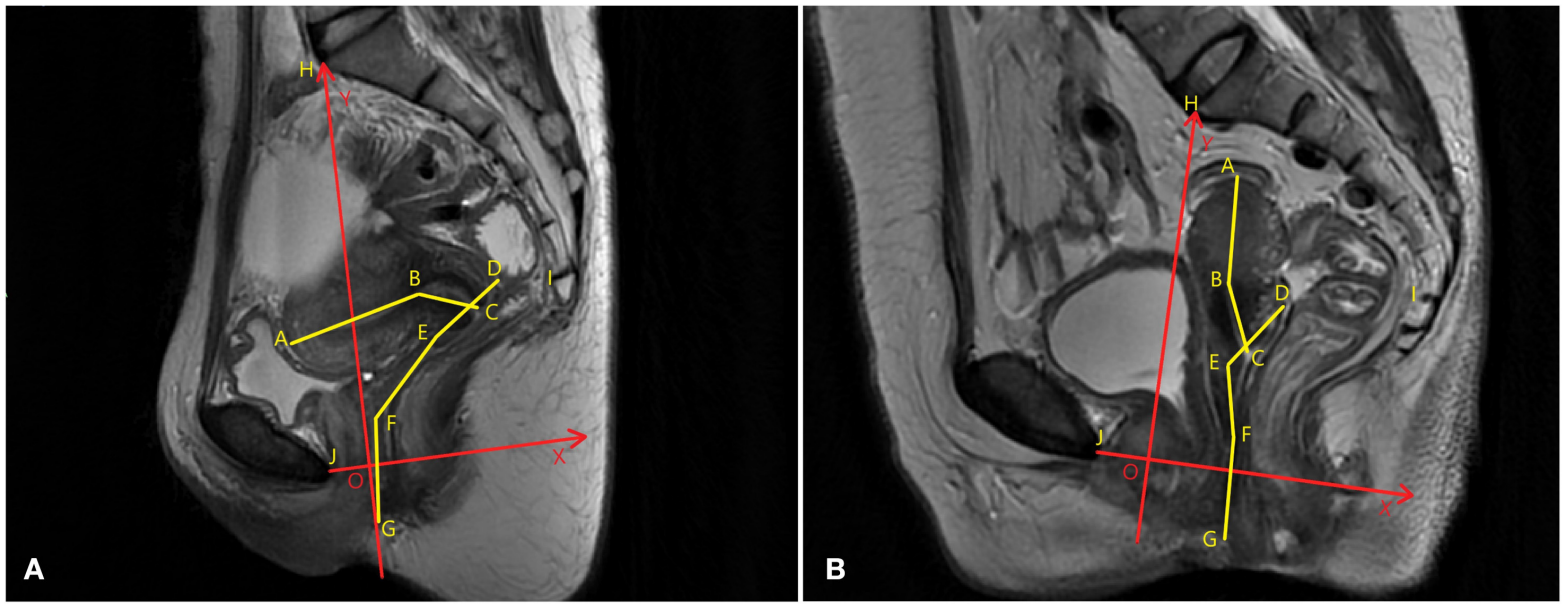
Uterine and vaginal analysis. (A) The central sagittal magnetic resonance (MR) image of a participant without prolapse is shown. (B) The sagittal median MR image of a participant with prolapse is shown. The sagittal plane analysis system of the axes, angles, and positions of uterus and vagina are established. To compare the shapes, positions, and angles of the uterus and vagina between different participants, a local coordinate system (XOY, in red) that quantifies the morphology was created. The OX axis was created by rotating the sacrococcygeal-inferior pubic point (SCIPP) line 34° clockwise. The OY axis is perpendicular to the X-axis through the sacral promontory, and the same coordinate system is used to compare the spatial position of each participant's uterus and vaginal axes.
